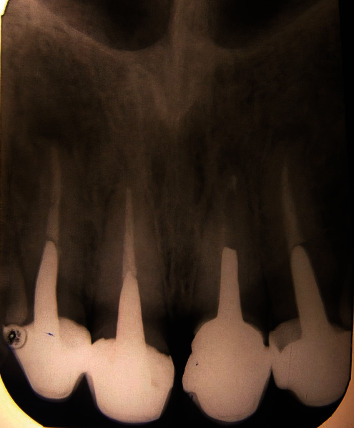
Radiographic view of patient's teeth.
